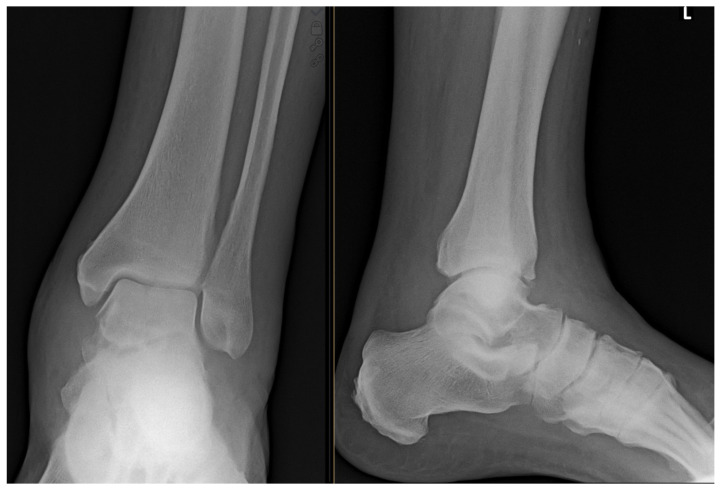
Initial X-ray image of the left ankle. The image shows normal picture with no detectable osteolytic lesions.
